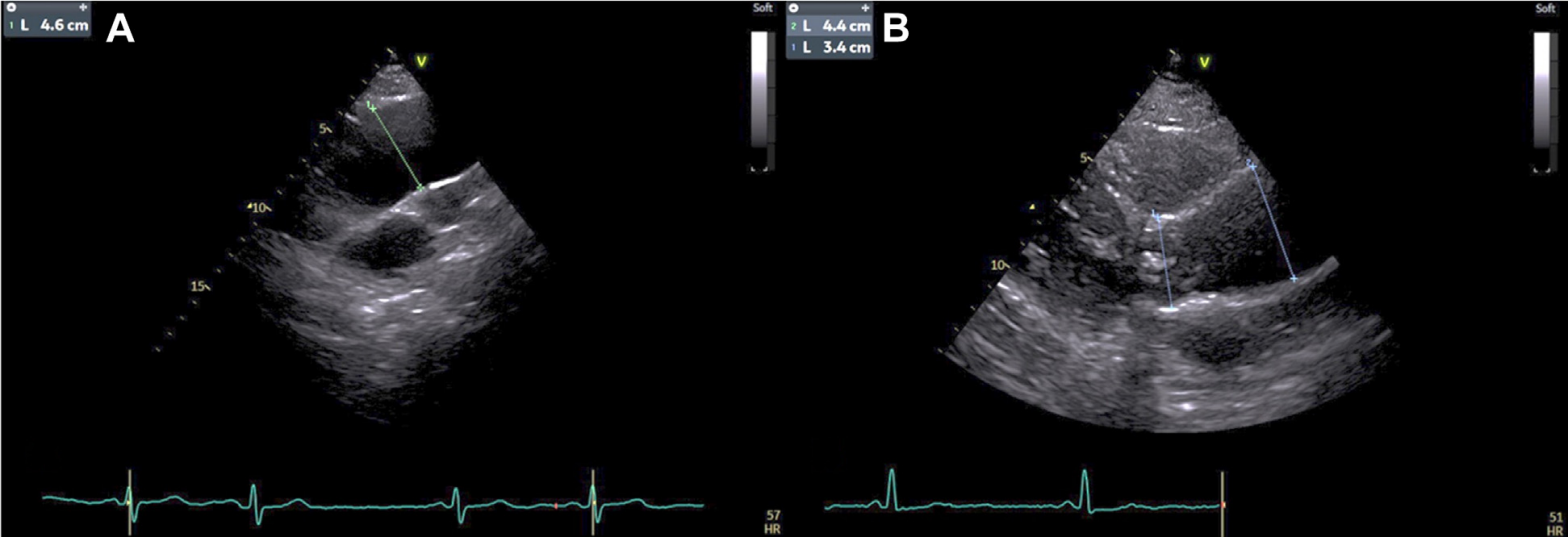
Ascending Aorta and Aortic Root Dilation The right parasternal view (B) allowed visualization of a more distal tract of the aorta vs parasternal long-axis view (A).
